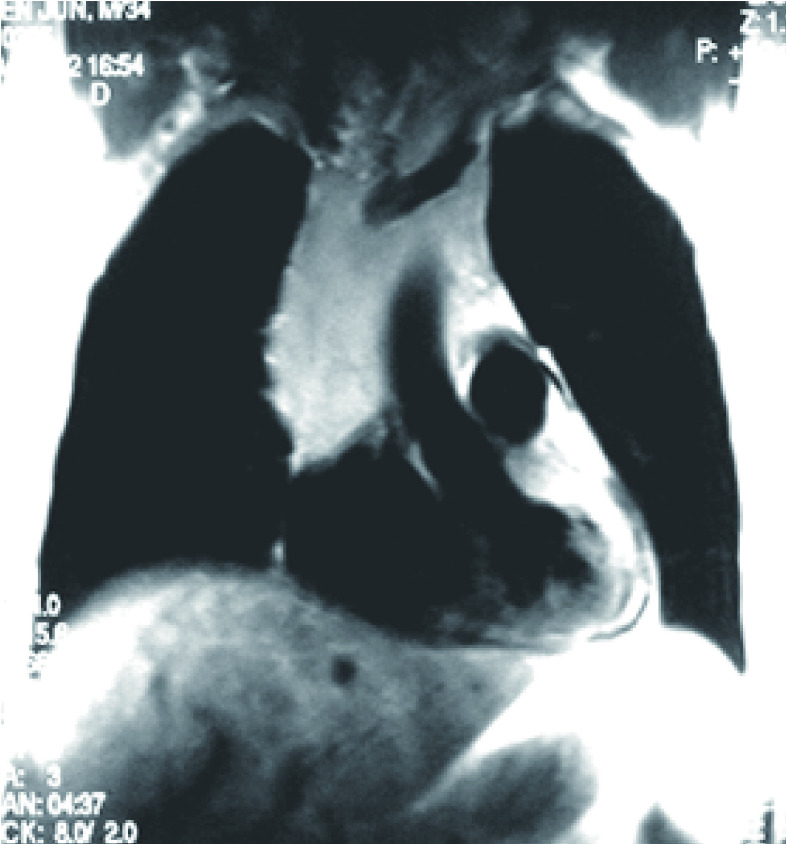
恶性胸腺瘤侵犯上腔静脉的MRI征象 MRI images of malignancy-related superior vena cava syndrome.MRI: magnetic resonance imaging.
